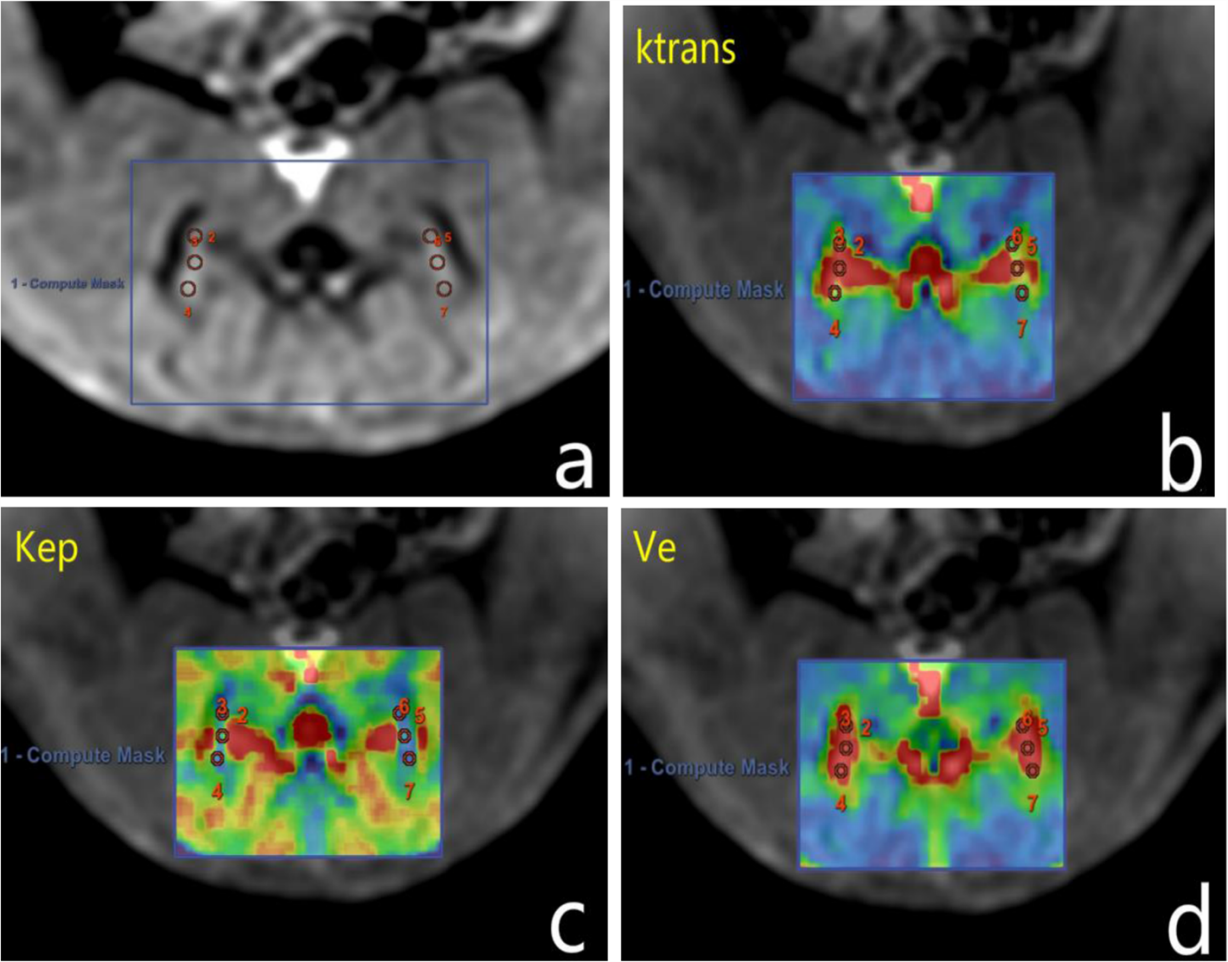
Representative images of ROIs outlined. a Image shows that ROIs have been manually drawn on magnified DCE-MRI image,and that the colour parametric maps of (b) K^trans^ (min^− 1^), (c) K_ep_ (min^− 1^) and (d) V_e_ are automatically generated
